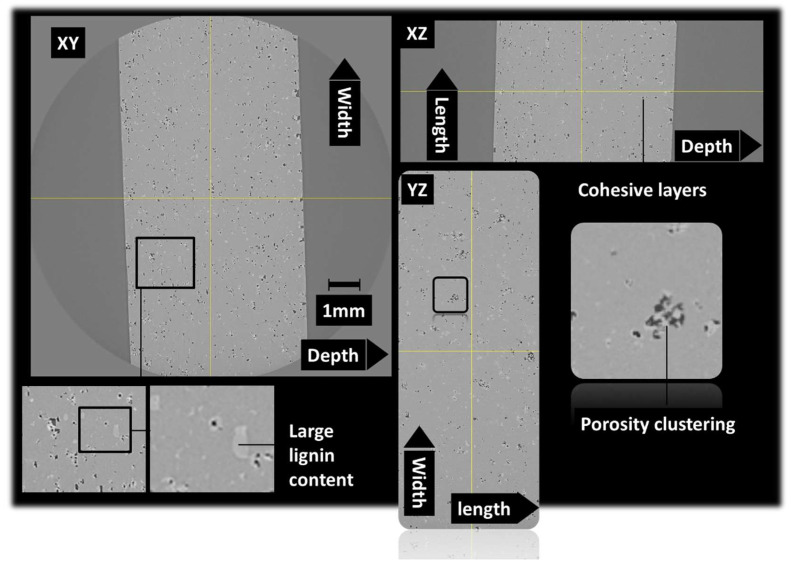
X-ray microtomography results: orthogonal views underlying the microstructure of 3D-printed cured acrylate-modified PLA /10 wt% lignin with an intense mixing stage.
